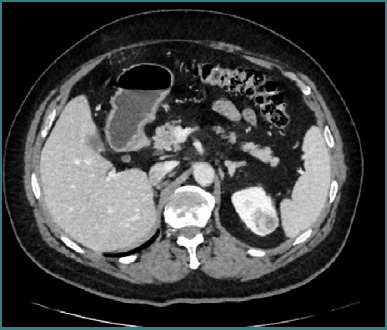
Follow-up computed tomography revealed the presence of another tumor at the level of the left upper renal pole
